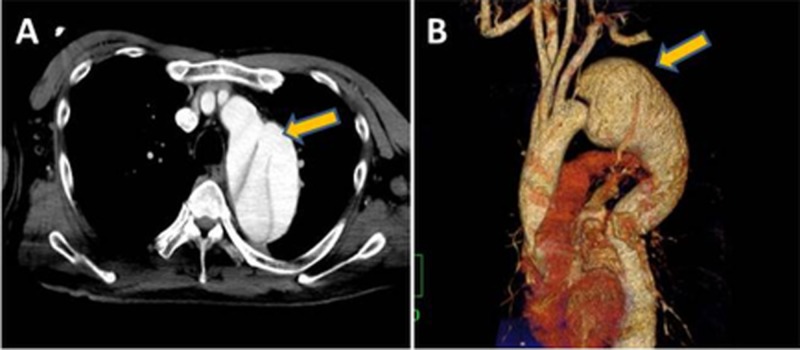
A-B. Preoperative computed tomography image: Enlargement of type B aortic dissection to 65 mm with a primary tear distal to the left subclavian artery
